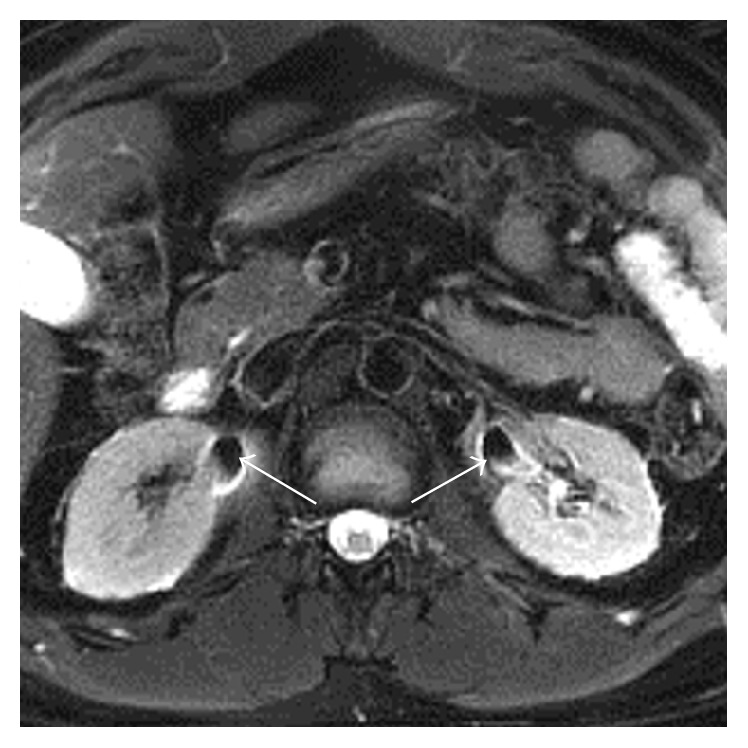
MRI artifacts mimicking kidney stones. An MRI image showing signal void due to T2^*∗*^ artifact from concentrated gadolinium in both collecting systems (arrows). These signal voids could readily be mistaken for stones.
